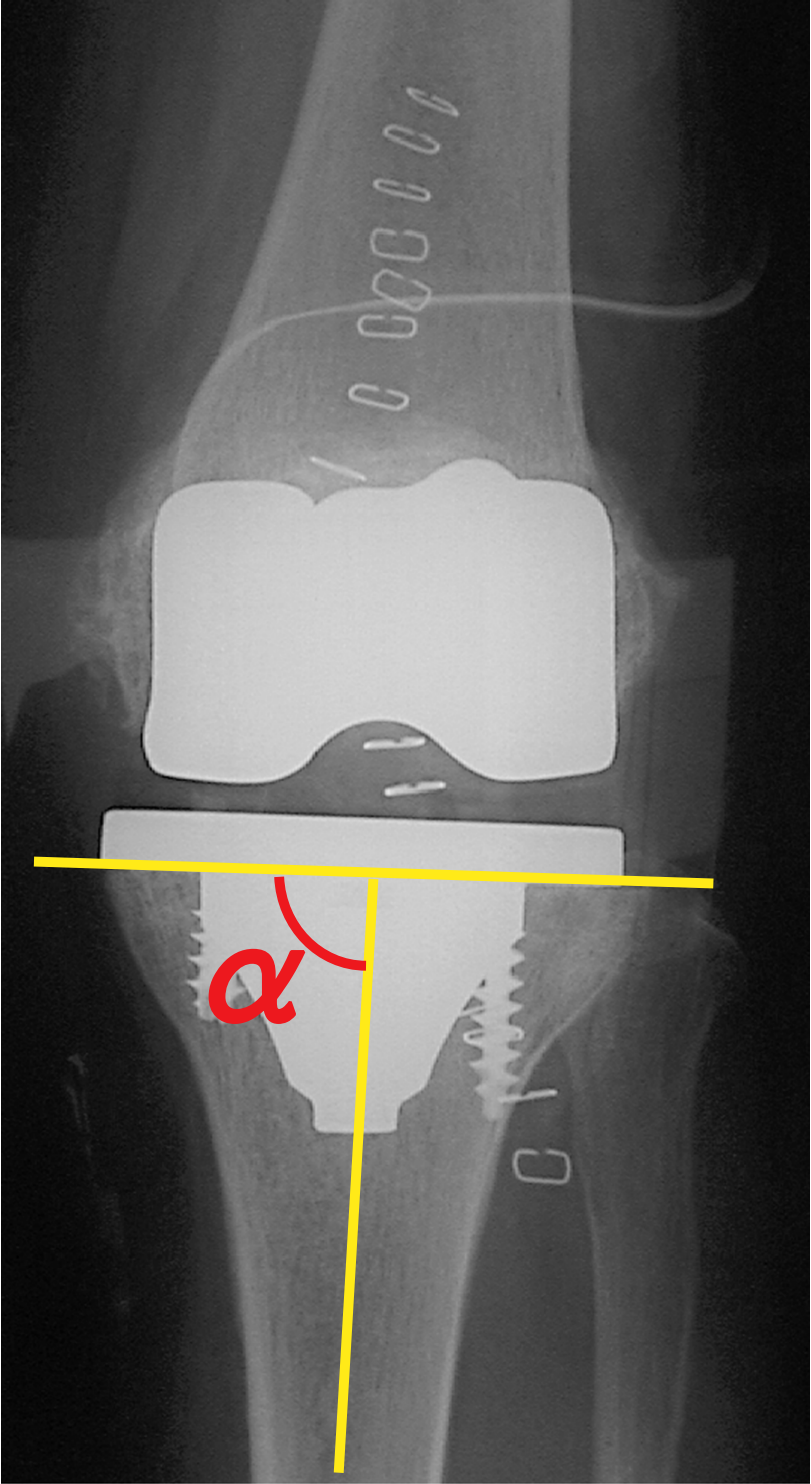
Anteroposterior radiograph of the knee. α: varus angle of the tibial osteotomy.
